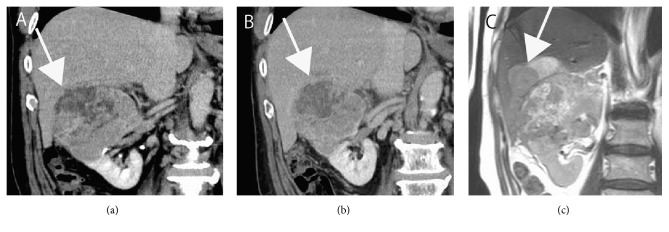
CT and magnetic resonance imaging (MRI) findings at the site of liver invasion*. Appearance of invasion front (arrows) is shown before axitinib treatment (a), 2 months after treatment (b), and 3 months after treatment (c). Focal invasion is suspected at pretreatment period (a); however, invasion was progressed at 2 months after treatment (b), and apparent nodular formation was observed at 3 months (c).
